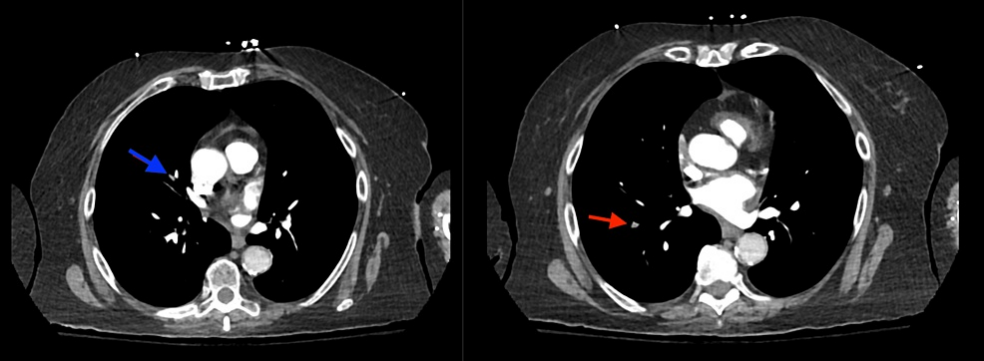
Computed tomography angiography of the chest A filling defect is noted in the bifurcation of the proximal segmental right middle lobe pulmonary artery and extends into the lateral subsegmental branch (blue arrow). A focal filling defect causing abrupt cutoff of the right lower lobe lateral basal subsegmental branch is also noted (red arrow).
